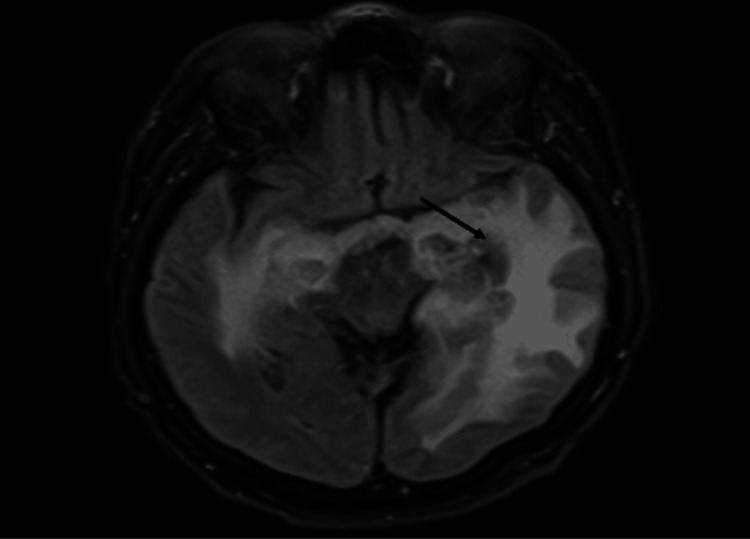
MRI from September 2022 showing edema in the mesial regions of both temporal lobes, with significant involvement of the hippocampi and periventricular distribution in left temporal lobe
